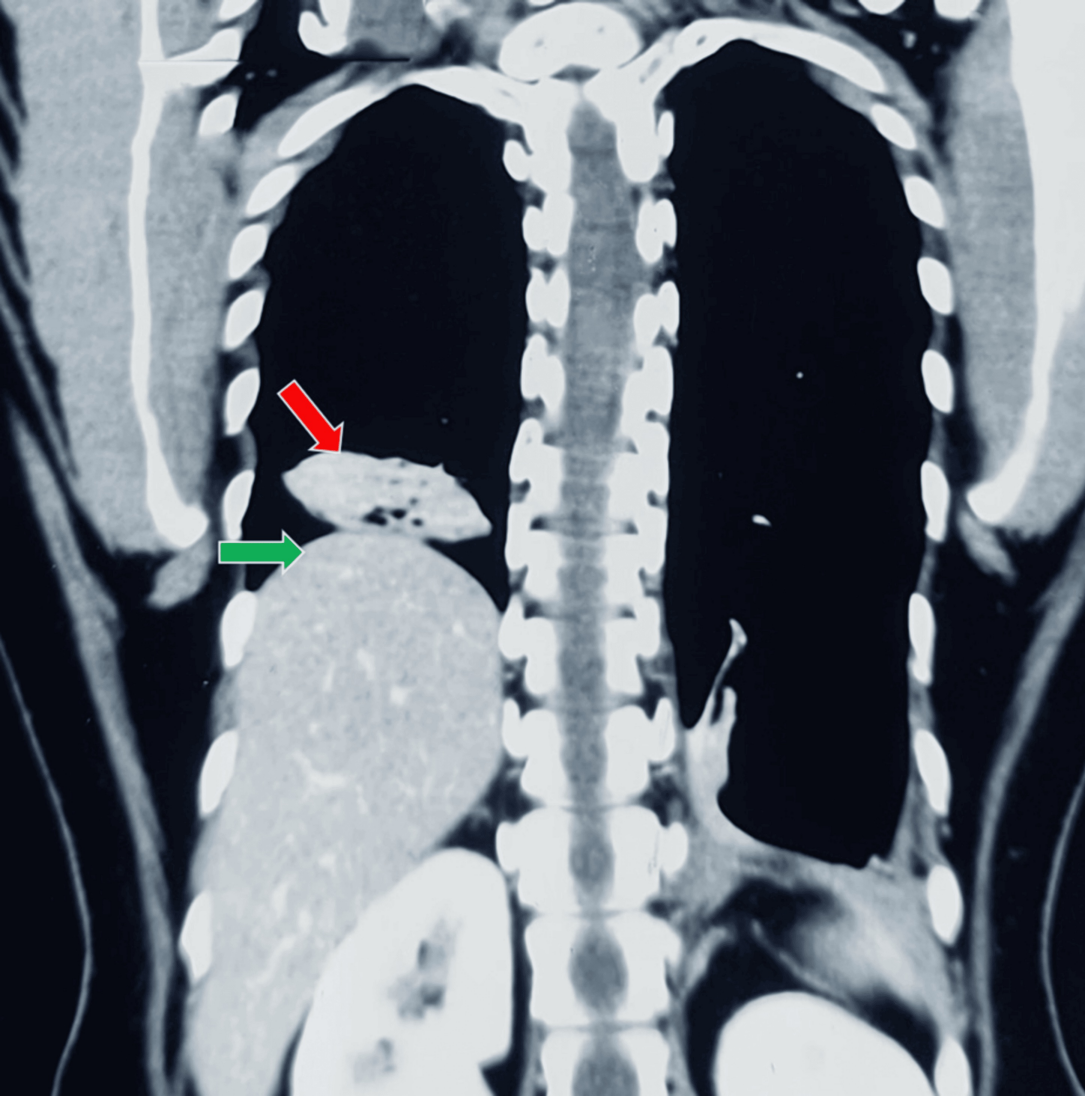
A CT scan of the chest showing an elevated right hemidiaphragm (green arrow), with a strange saucer-shaped lesion (red arrow) above it resembling an unidentified flying object (UFO).
